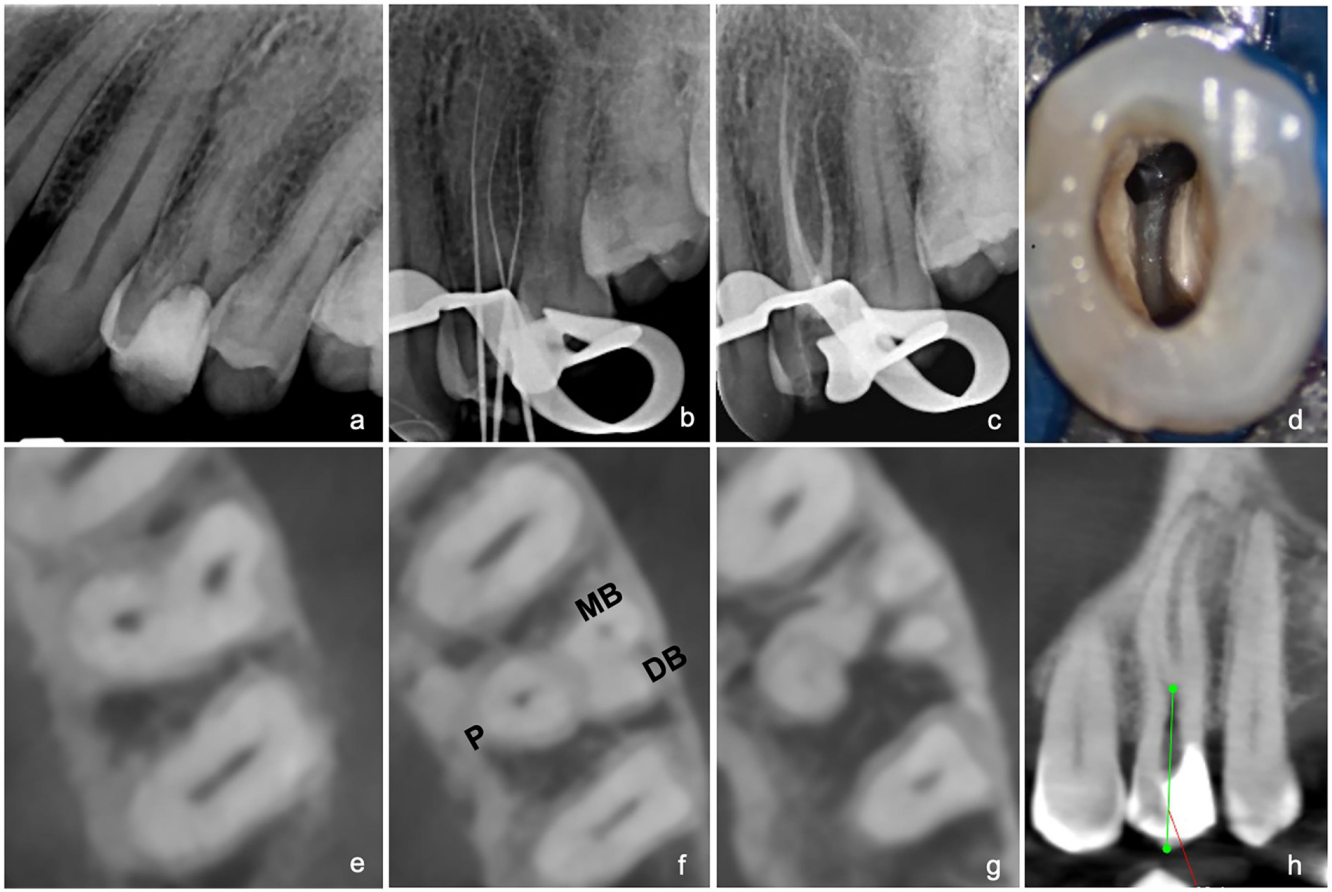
(a) Preoperative periapical radiograph of tooth #24. (b) Radiographic working length determination. (c) Postoperative radiograph confirming obturation. (d) Clinical view of pulp chamber showing buccal canal orifices. (e) Coronal CBCT view showing buccal and palatal canals. (f) Axial CBCT view at midroot level showing buccal canal bifurcation. (g) Axial view showing three separate roots at midroot level. (h) Sagittal CBCT view showing bifurcation of buccal canal at 12.4 mm.
